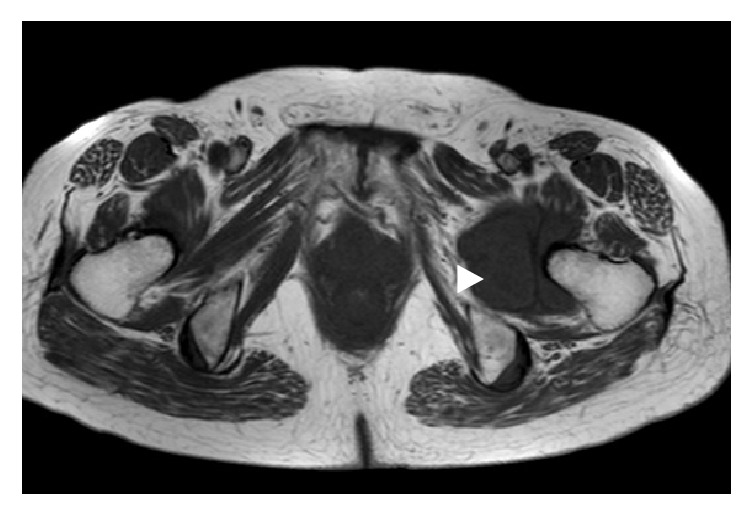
T1-weighted axial magnetic resonance image of the left hip joint. The extra-articular herniation sac is indicated by the arrowhead.
